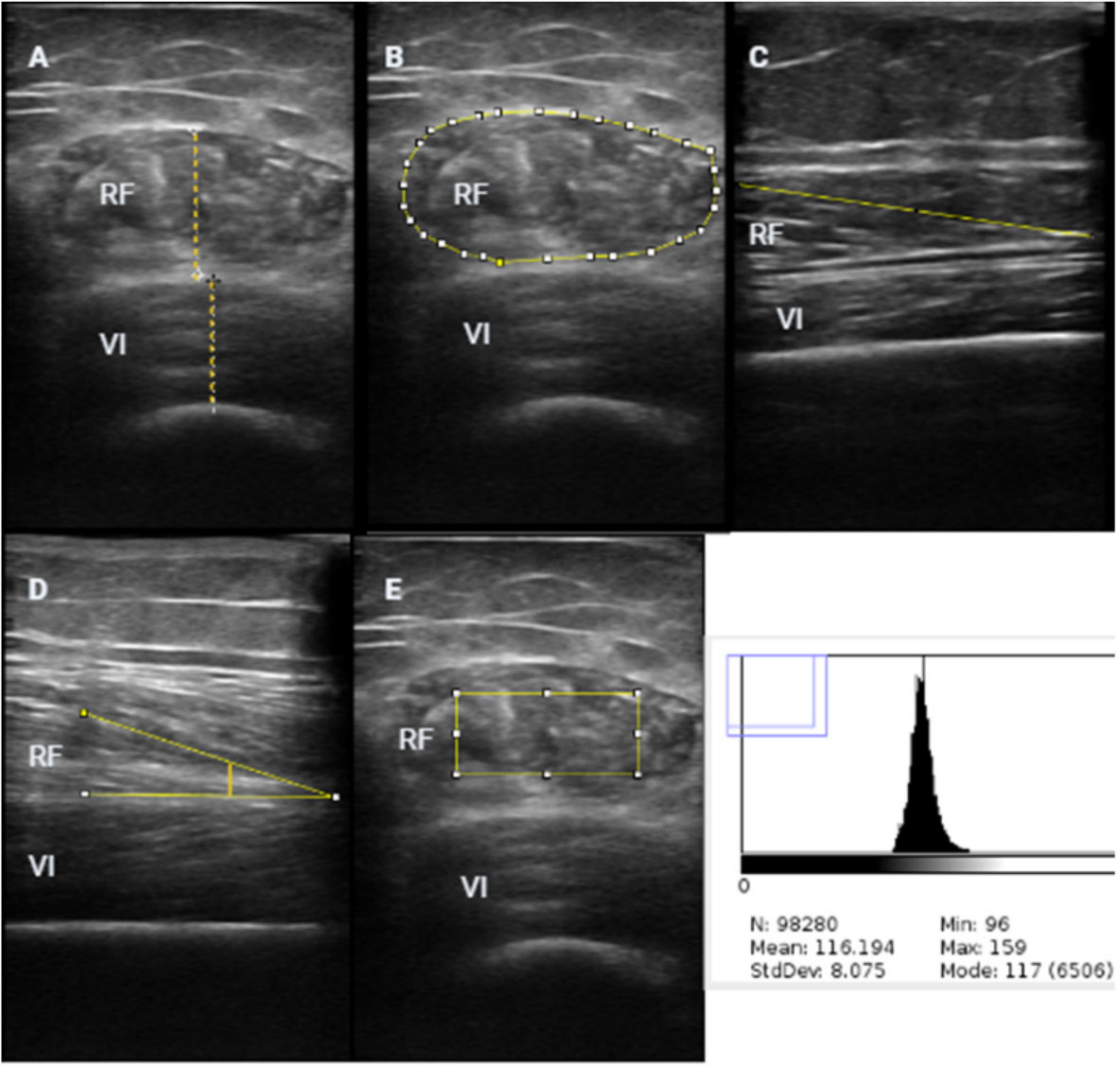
Body composition assessment by quadriceps muscle ultrasound (US). (A) Rectus femoris and vastus intermedius muscle thickness. (B) Rectus femoris cross-sectional area. (C) Fascicle length. (D) Pennation angle. (E) Echogenicity evaluation.
